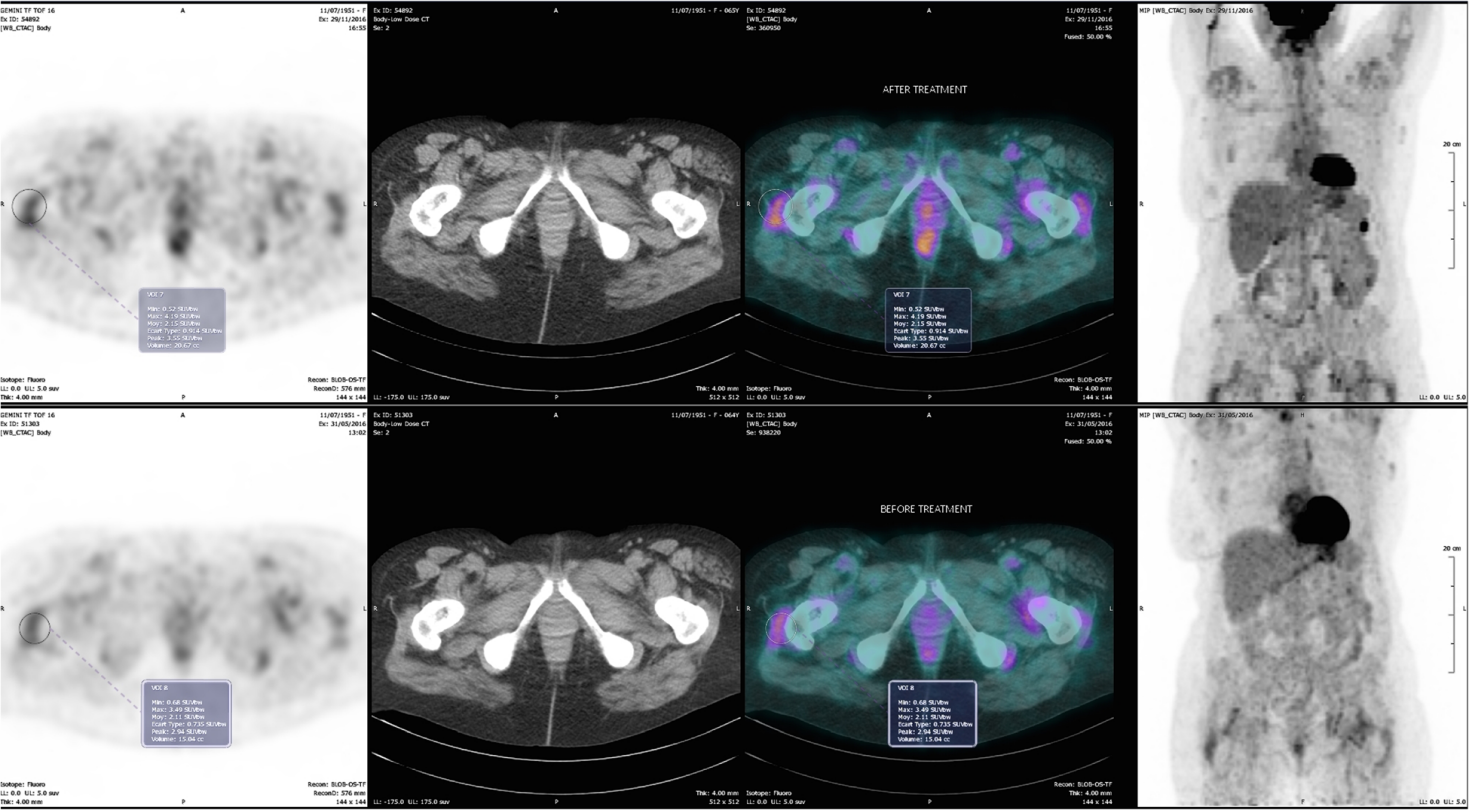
PET scan: upper image: after treatment; lower: before treatment. Shoulders. Diffuse, metabolically active deposits, specifically in the settings of pain. The pattern is consistent with diffuse periarticular hyperfixation. The SUV increases from 2.99 to 4.02 at 6 months
